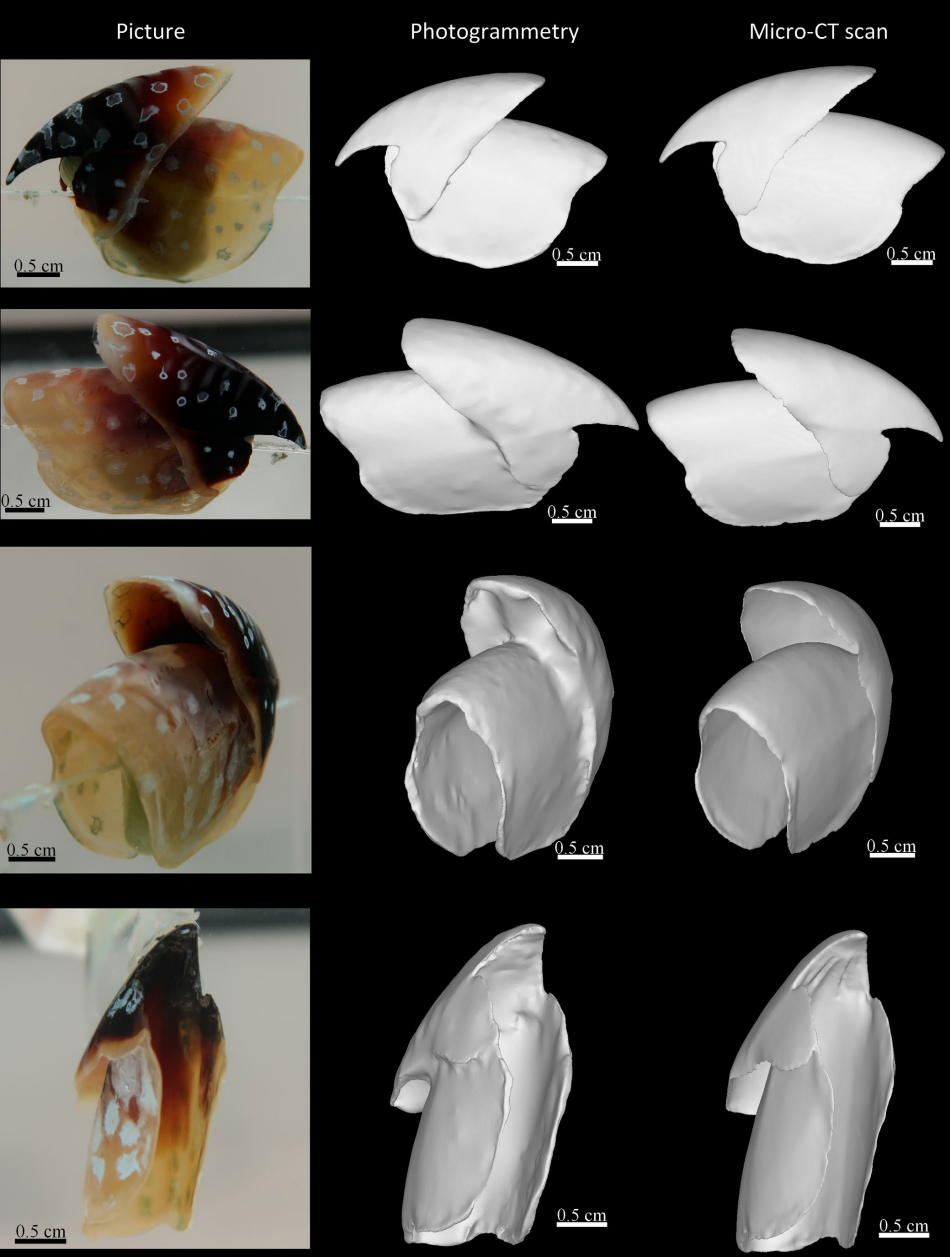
Comparison between a photogrammetric model of an upper beak of *Ommastrephes cylindraceus* and the same reconstruction using micro‐CT scanning. top: lateral left view; middle: lateral right and posterior views; bottom: ventral view. Bottom picture is upside‐down to conserve the conventional orientation of beaks
